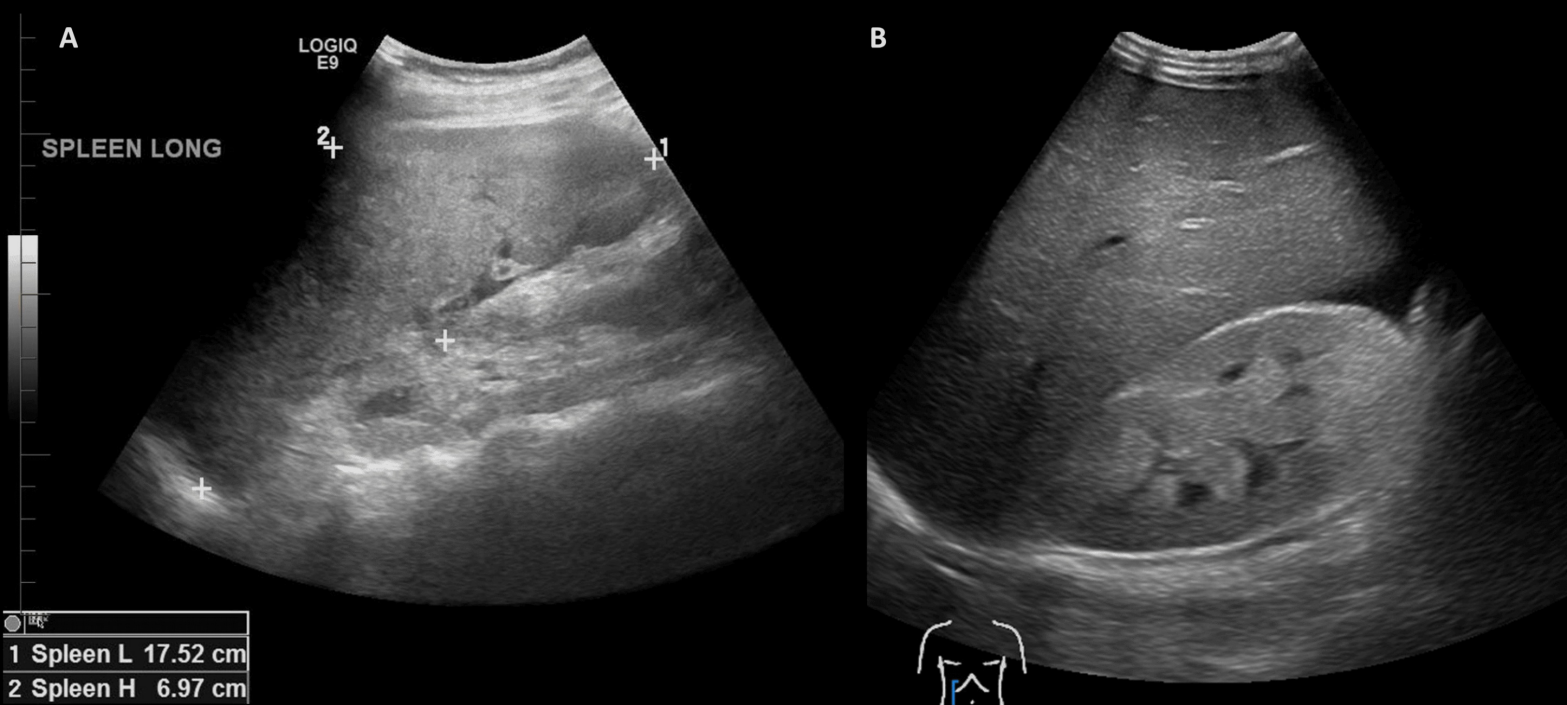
Abdominal ultrasound Abdominal ultrasound with corresponding symptoms seen in HLH. Images show splenomegaly (A) and hyperechoic kidneys (B). HLH: hemophagocytic lymphohistiocytosis
